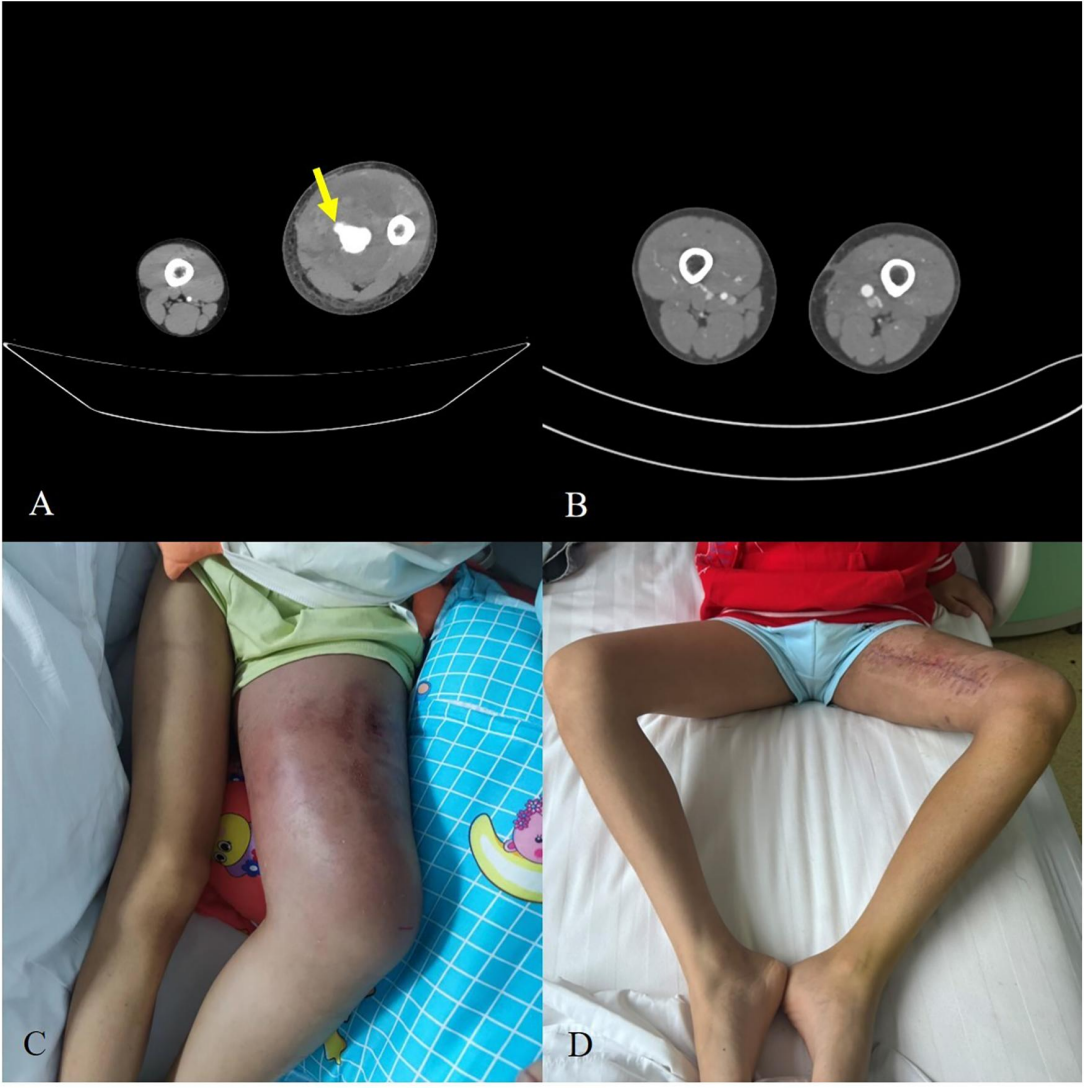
(A) Preoperative CTA demonstrating contrast extravasation from the pseudoaneurysm (arrows). (B) Postoperative CTA showing restored vessel patency. (C) Clinical photograph of preoperative thigh swelling. (D) Postoperative recovery with significant reduction of swelling and healed incision.
